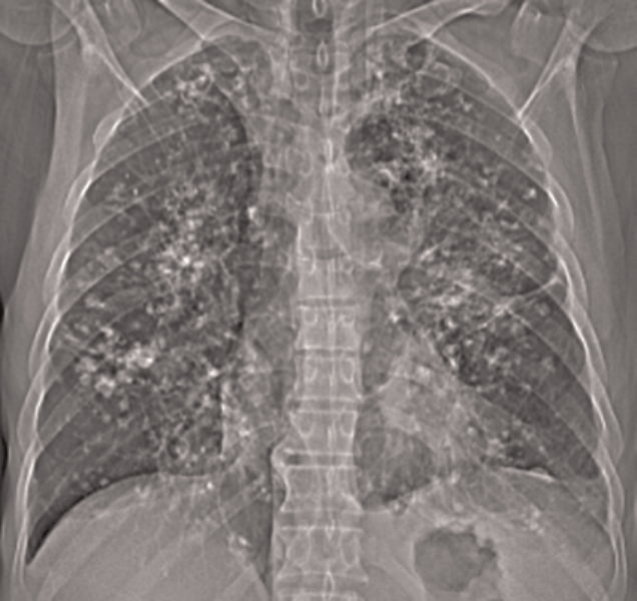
Chest radiograph demonstrating diffuse pulmonary opacities with extensive foci of calcification.
